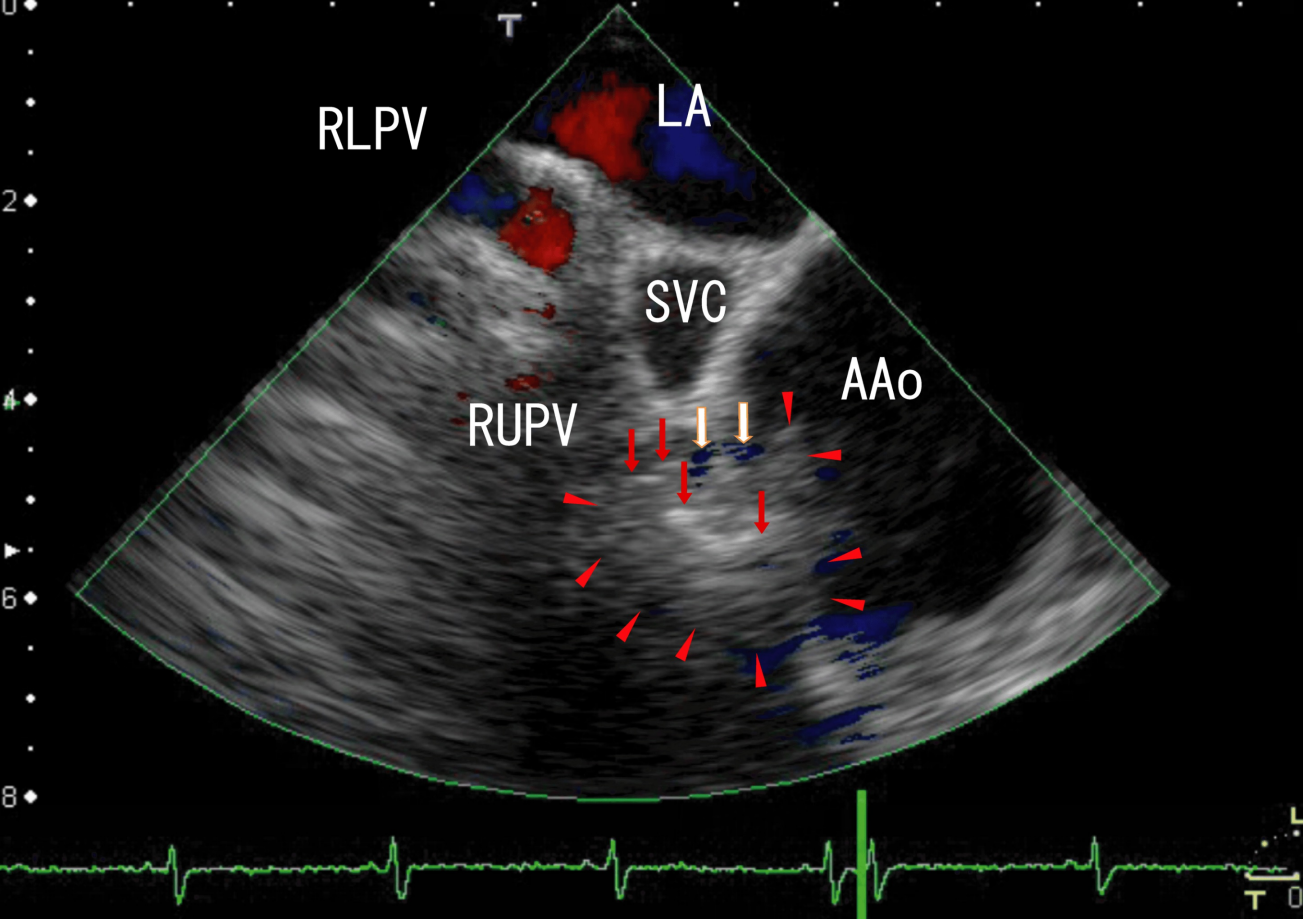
TEE images showing AAo thrombi and linear dark-white thrombi The TEE revealed thrombi in the AAo and linear dark-white thrombi extending from the RUPV into the AAo (red arrows), which had lost some clear white areas. Shorter linear white thrombi were observed in a similar position in Figure 1, which were situated in the mass on the AAo wall (arrowheads). Small blue areas were present between the AAo wall and the linear white thrombi on the AAo wall (white arrows), indicating a cleft in the AAo wall and penetration of the AAo wall by the thrombi. Approximately 2 cm of the right anterior AAo wall appeared to have lost its normal structure. A large mass (1.5 × 1.5 cm) was present on the AAo wall. The red areas in the LA indicate blood flow from the RUPV. TEE: Transesophageal echocardiography; AAo: Ascending aorta; LA: Left atrium; RLPV: Right lower pulmonary vein; RUPV: Right upper pulmonary vein; SVC: Superior vena cava.
